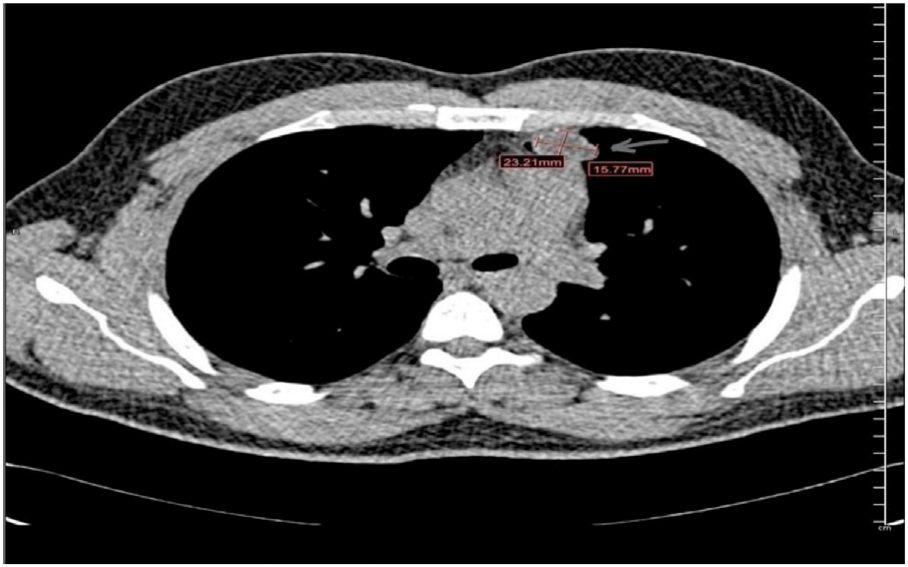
CT scan of the chest with contrast, demonstrating an anterior mediastinal hypodense mass 23.2 × 15.7 mm in size.
